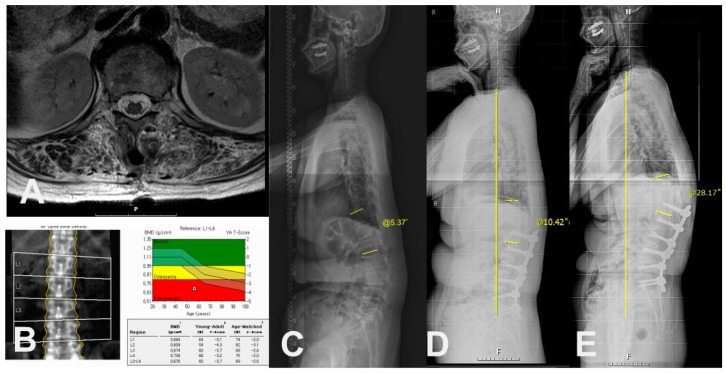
Case of 66-year-old female patient with PJK. (A) Preoperative axial T2-weighted magnetic resonance image showing erector spinae muscle with severe (>50%) fat infiltration at T12-L1 level. (B) Preoperative BMD showing osteoporosis. (C) Preoperative whole spine standing lateral radiograph showing PJA of 5.37°. (D) Postoperative 3-month standing lateral whole spine radiograph showing PJA of 10.42°. (E) Postoperative 2-year standing lateral whole spine radiograph showing PJK (PJA of 28.17°) with increased C7SVA.
